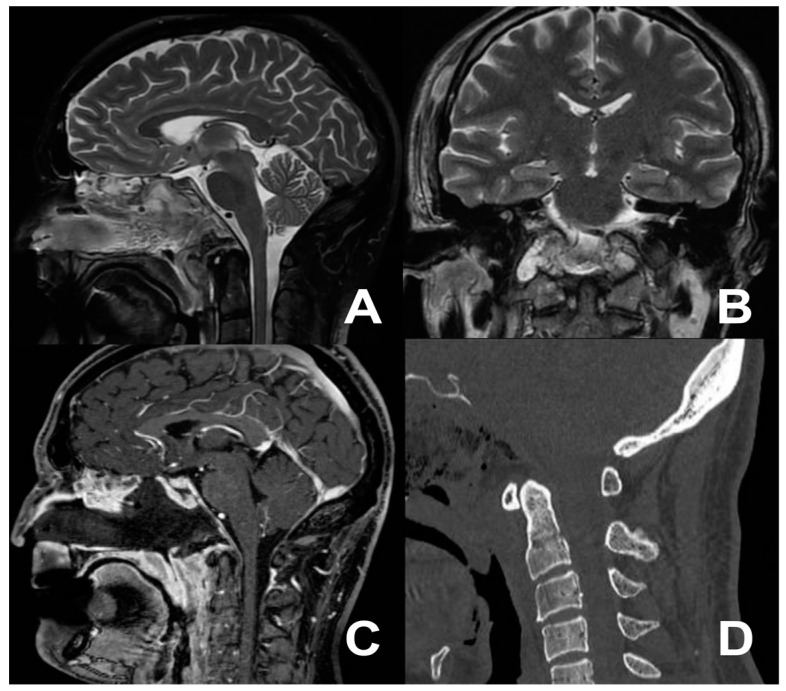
Postoperative MRI (A–C) and CT scan (D) showing the extent of tumor removal.
